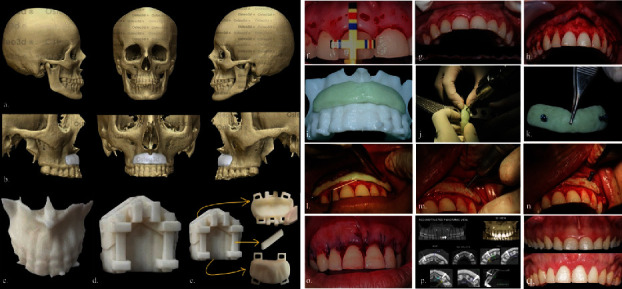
Digital planning: (a) computed tomography (CT) scan; (b) virtual planning to plan position, size, and shape of PMMA block; (c) prototype of cropped maxillary segment for test fit; (d) prototype of mould for fabrication of PMMA block; (e) parts of 3D printed mould. Surgical intervention: (f) bleeding points are marked using Chu's aesthetic gauge; (g) gingivectomy is performed; (h) full thickness flap elevated; (i) passive adaptation of PMMA block checked by placing over printed maxillary segment; (j) screw positions are marked, and two holes are drilled of 2 mm diameter using bone drills; (k) extraoral placement of titanium screw over the prepared PMMA block; (l–m) PMMA block placement; (n) fixation of PMMA block using titanium screws; (o) flap adaptation using sling sutures; (p) postoperative CBCT to confirm the position of PMMA block; (q) pre- and postoperative intraoral photographs.
